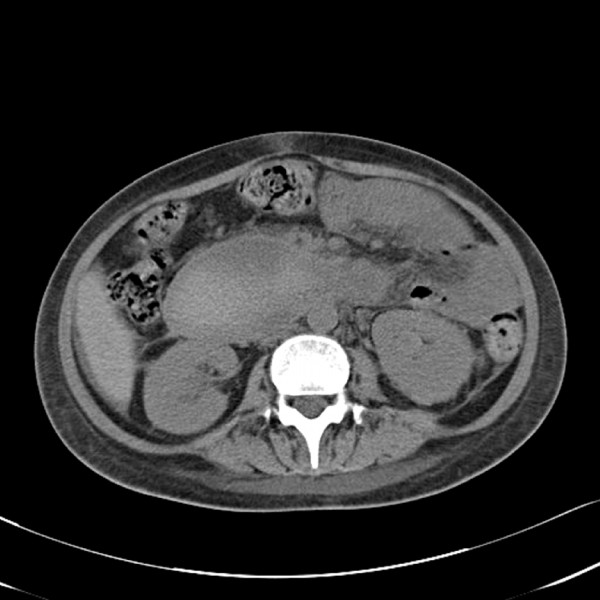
Follow-up CT of the abdomen 4 weeks post-endoscopy demonstrates partial regression of the hematoma.
